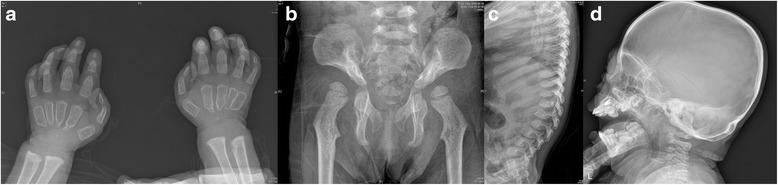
Radiographs of 21-month-old Korean girl with ML II. a Radiograph of hands and wrists showing broad and under-modelled proximal phalanges (*bullet-shaped*) and proximal pointing of metacarpals. b Radiograph of pelvis and proximal femurs showing dysplasia/resorption of the lower third of the ilia femoral heads and femoral necks and e. Clierd’s crook deformity.” c Radiograph of spine showing thoracolumbar kyphosis and beaking of the vertebrae. d Radiograph of skull showing J-shaped sella turcica
